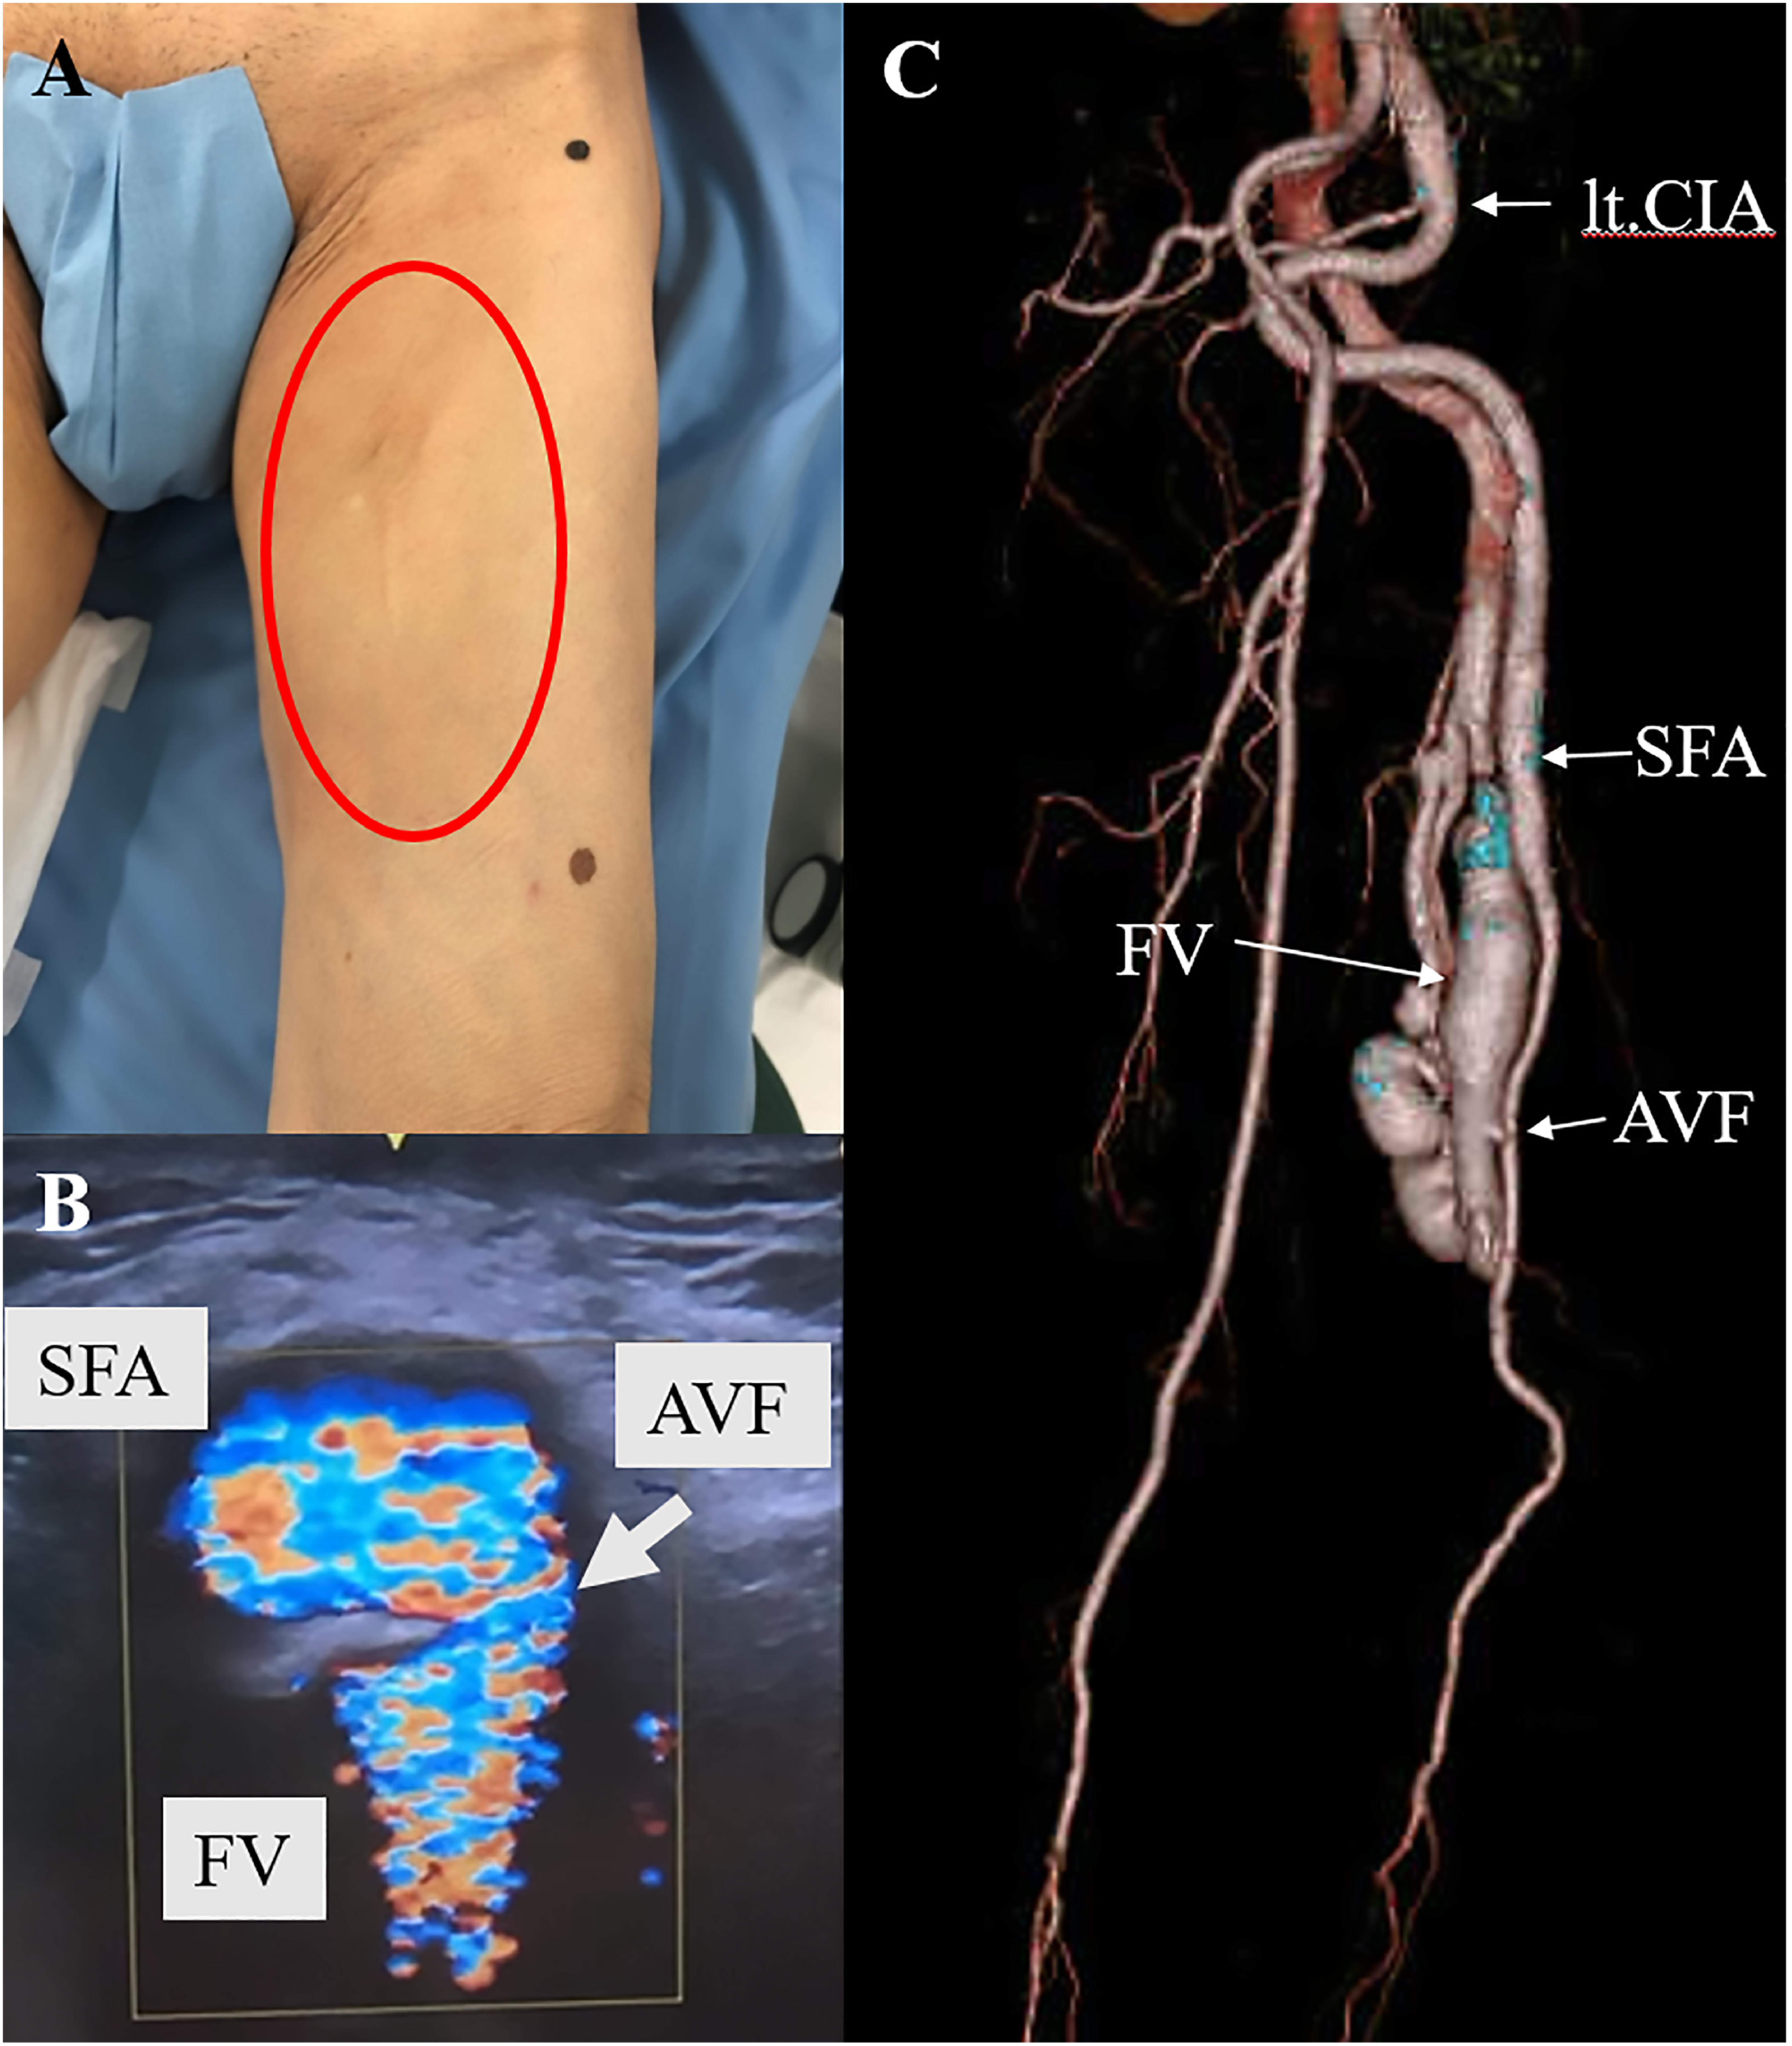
Fig. 1 Preoperative examination. (A) Preoperative condition of the upper extremity. (B) Echography shows the femoral arteriovenous fistula (AVF). (C) Preoperative computed tomography angiography shows AVF and dilation of the femoral vein (FV). Dilation was also observed from the common iliac artery (CIA) to the superficial femoral artery (SFA).
